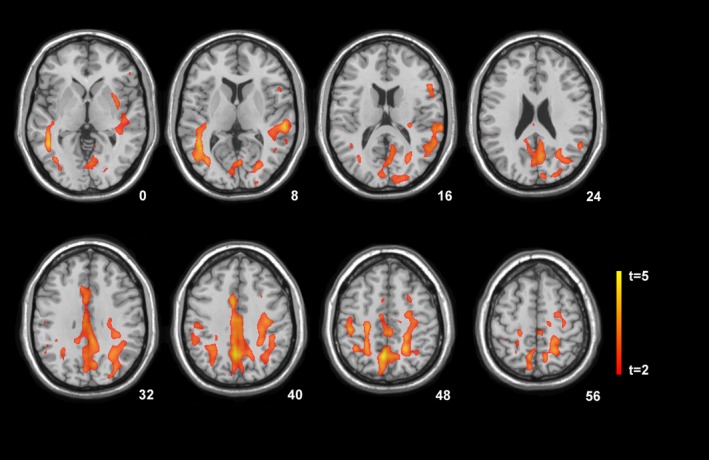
Statistical parametric mapping (*t*) axial intensity projection maps rendered onto a stereotactically normalized MRI scan, showing areas of significant increases in the transverse relaxation rate (R2*) in a cohort of patients with subarachnoid hemorrhage versus healthy control subjects 12 months after bleeding (color code, yellow to orange). The number at the bottom right corner of each MRI scan corresponds to the *z* coordinate in Montreal Neurological Institute space.
